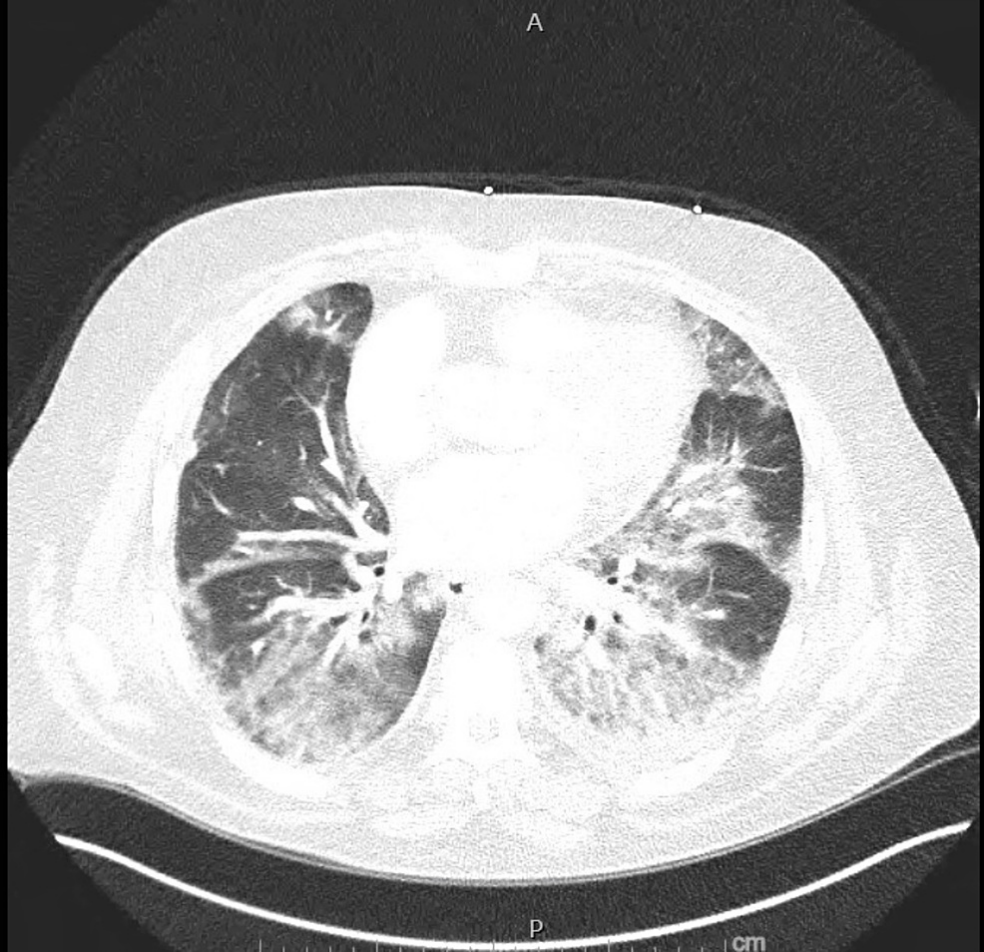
CT angiography of the chest This was performed due to elevated D-dimer levels to rule out a pulmonary embolus. There was no evidence of pulmonary embolism, but it showed diffuse bilateral lung infiltrates, similar to the findings on the chest X-ray.
